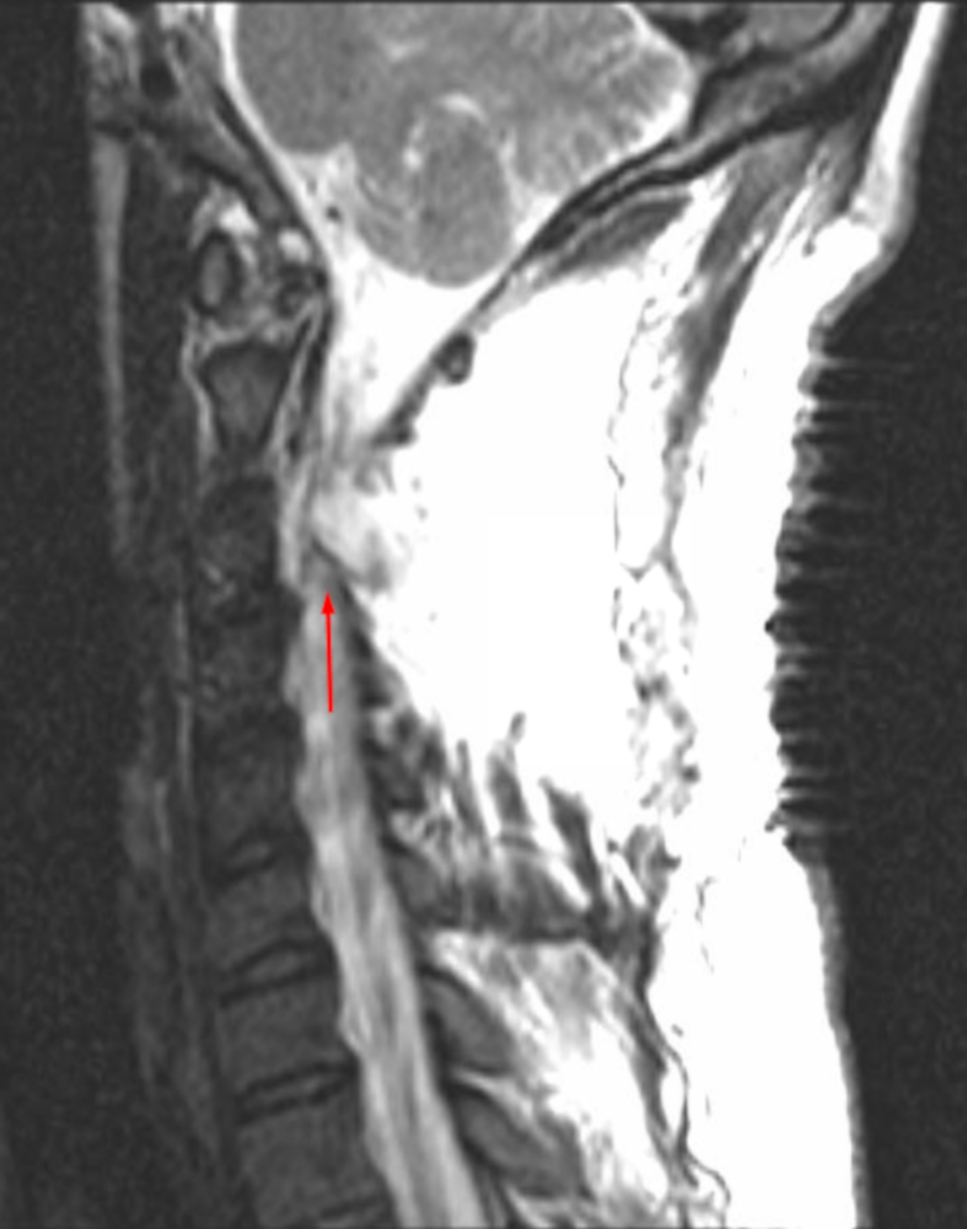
Cervical spine sagittal MRI without contrast demonstrating a large pseudomeningocele within the surgical site prior to dural repair with BMP MRI (Magnetic Resonance Imaging), BMP (Bone Morphogenetic Protein)
